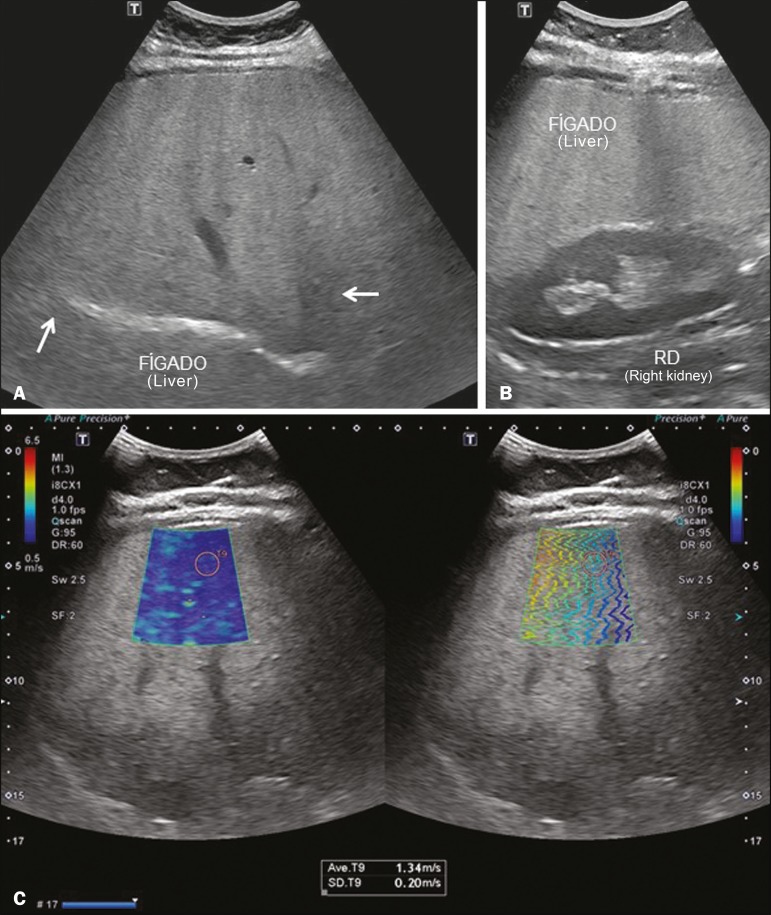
A: Ultrasound examination of a liver with moderate steatosis, as characterized by increased echogenicity of the liver parenchyma and posterior attenuation of the ultrasound beam. The attenuation of the ultrasound beam decreases the definition of the hepatic vessels and the diaphragm, although they are still identifiable (arrow). The echogenicity of the liver is higher than is that of the right kidney (B). C: A 2D-SWE study showing greater parenchymal stiffness, albeit still within normal limits (1.34 m/s), consistent with a METAVIR stage of F0/F1. Although the elastography map is a bit less homogeneous, it is still predominantly blue, indicating less stiffness, despite a larger number of green areas.
